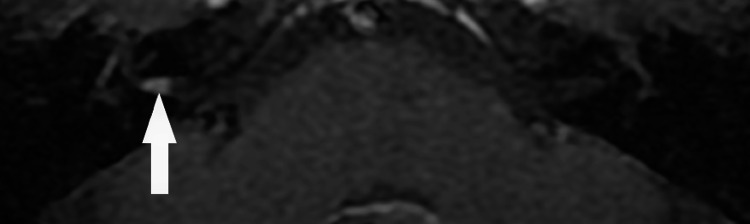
Axial T1 post-contrast magnetic resonance image with fat saturation of the same patient demonstrating enhancement (arrow) in the right internal auditory canal consistent with a very small vestibular schwannoma.
